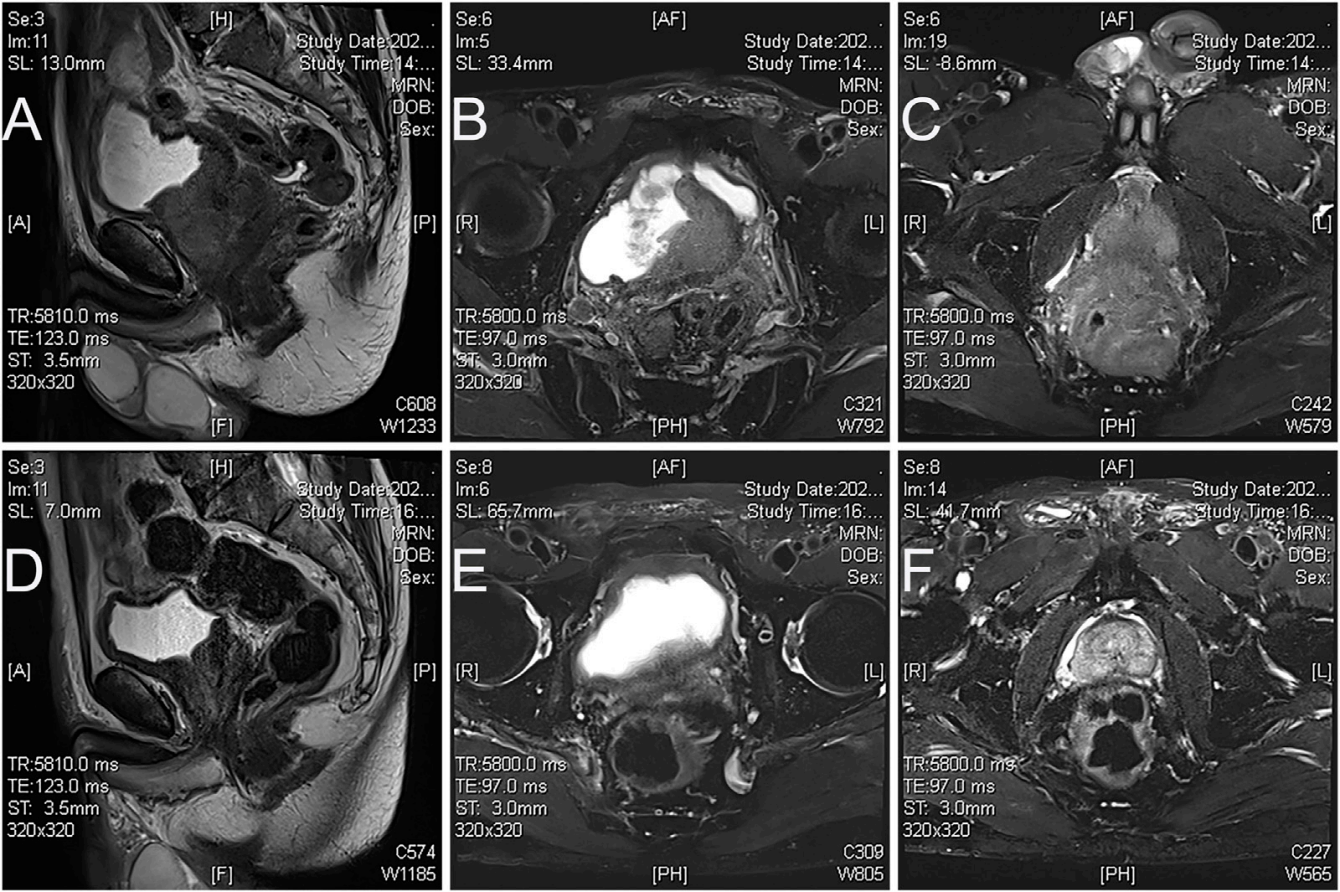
Magnetic resonance images of apalutamide before and 3 months post neoadjuvant treatment in case 2. (A), sagittal view, (B,C), axial view T2 imaging showed that prostate cancer broke through the prostate capsule and invaded the rectum, bladder neck, and seminal vesicles before neoadjuvant treatment; (A), sagittal view, (B,C), axial view T2 imaging showed that prostate cancer retracted significantly after neoadjuvant therapy (D), the boundary between the prostate and rectum seemed more straightforward, with no apparent tumor involvement in the bladder neck, but lesions invaded seminal vesicle still exists (E,F).
